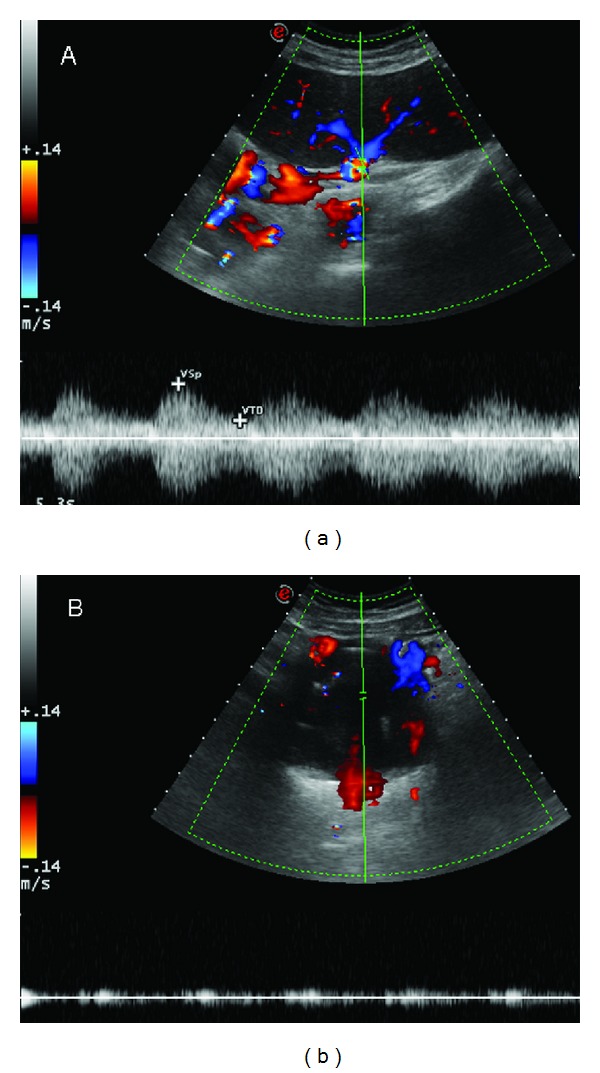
Abdominal ultrasound confirming the exclusion of the SAAs with complete preservation of distal flow to the spleen.
